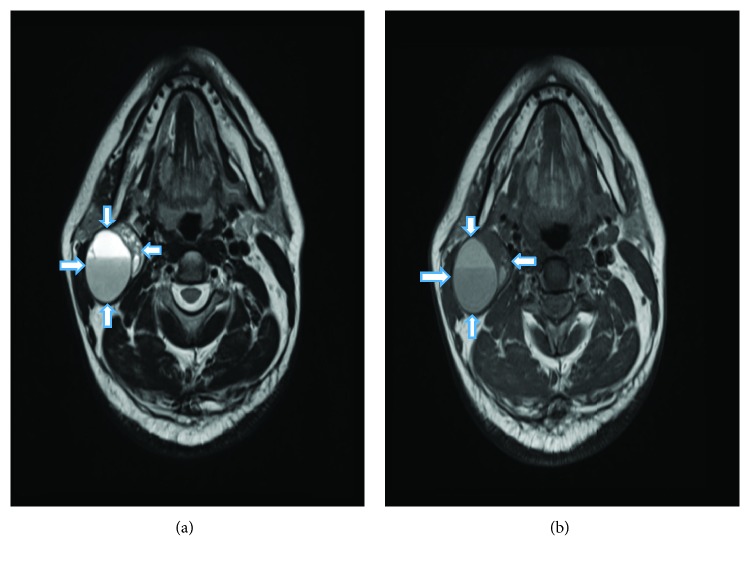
(a) T2-weighted magnetic resonance imaging shows hyperintense fluid-fluid level and isointense eccentric solid area (arrows showing edge of the tumor). (b) T1-weighted magnetic resonance imaging shows hyperintense fluid-fluid level (arrows showing edge of the tumor).
